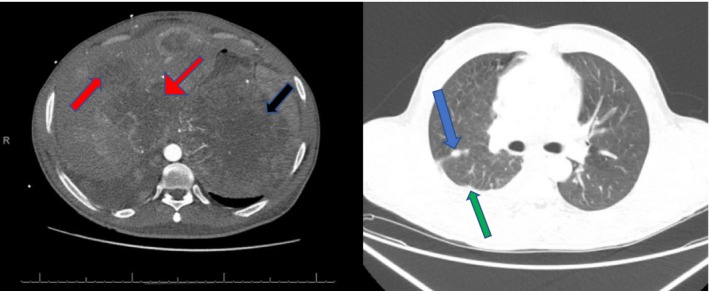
CT images showing lung metastasis (blue arrows), pleural‐based metastatic nodule (green arrow), large liver metastasis (red arrows), and a large approximately 10 × 9 cm left renal mass (black arrows)
